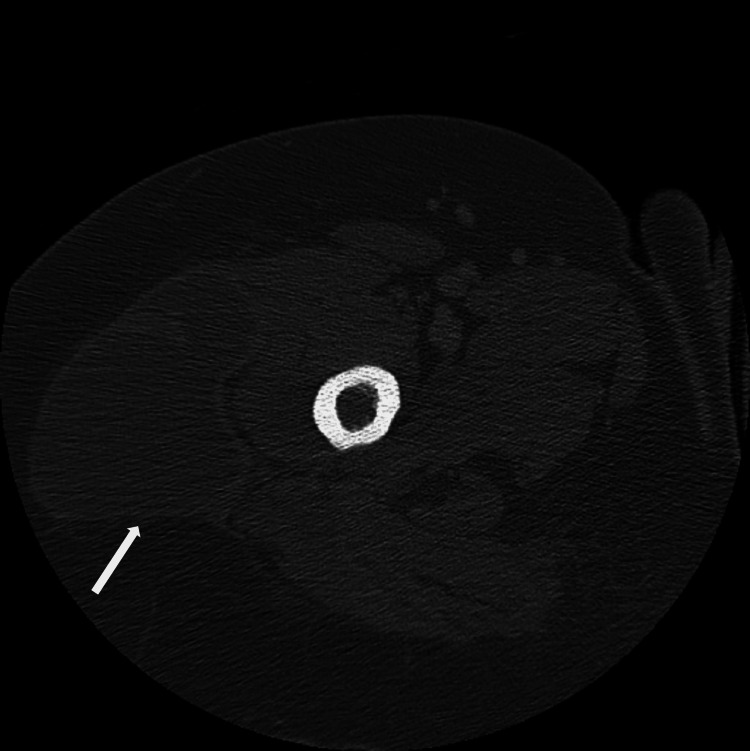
A CT of the right femur with contrast at a subsequent ED visit demonstrated large fluid collection findings similar to previous studies.
